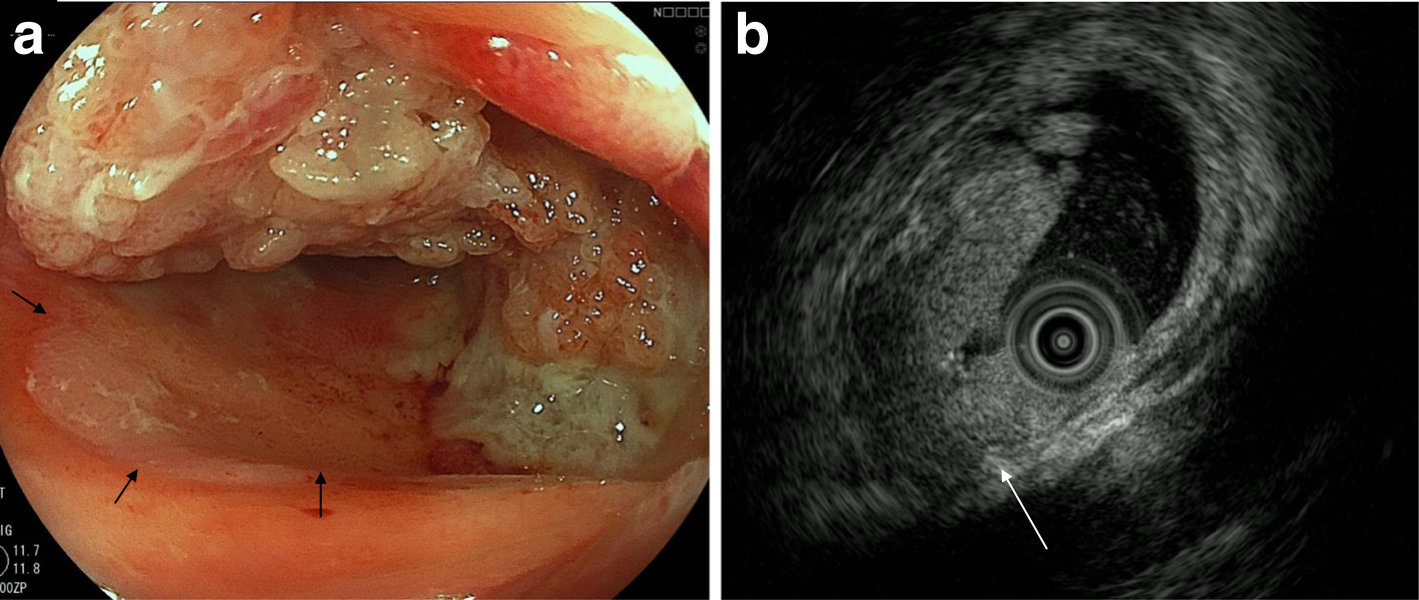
a Transvaginal endoscopy. Anastomosis of ectopic sigmoid colon with residual vagina (black arrows). A 45-mm protruding lesion was observed in the vicinity of the anastomosis site, occupying about three quarters of the lumen. b Transvaginal ultrasonography. Muscularis propria layer (hypoechoic fourth layer) was interrupted with an unclear layer pattern. Hypoechoic tumor appeared to invade beyond the colon wall (white arrow)
